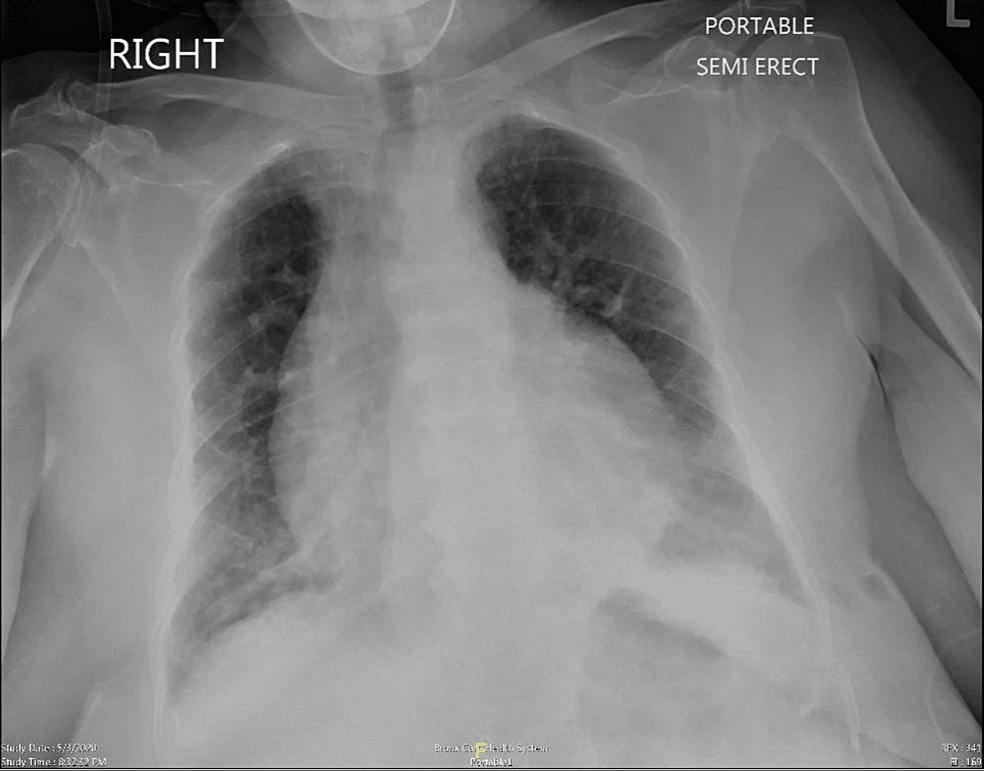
Chest X-ray with a bottle-shaped cardiac silhouette
